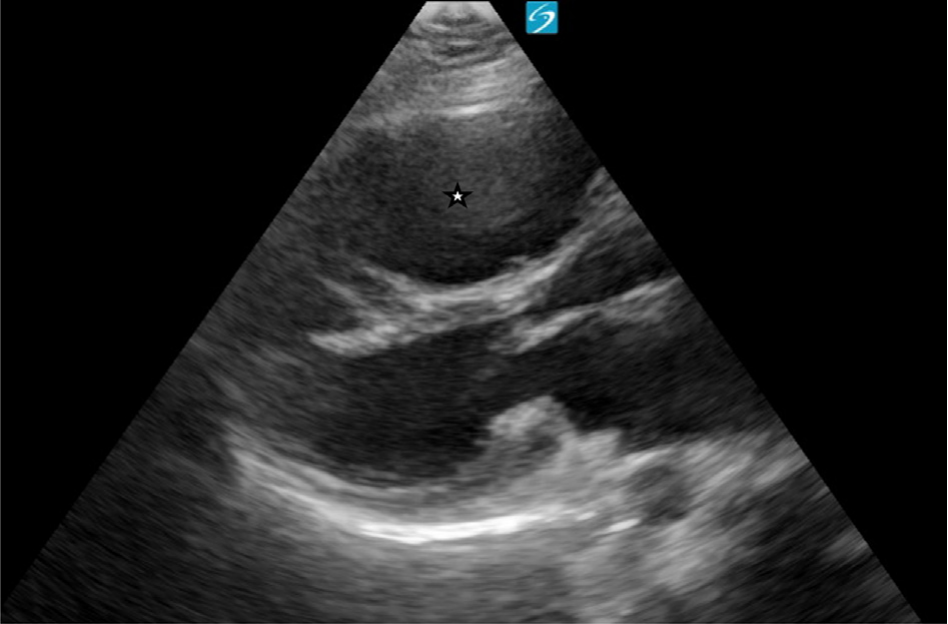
Point-of-care ultrasound (parasternal long-axis view) demonstrating a dilated right ventricle. Image taken by the author.
